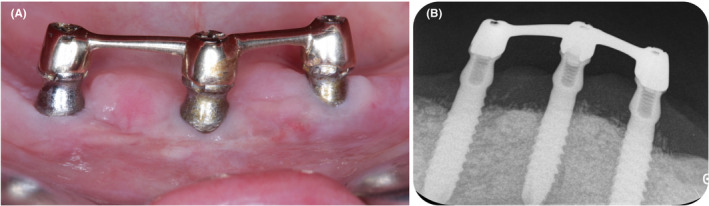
A) Clinical view; B) Radiographic examination 1 y after surgical treatment
